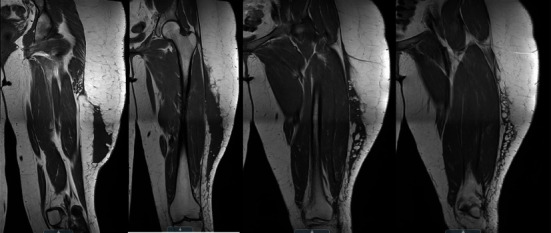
MRI T1 sequence of the left thigh approximately 1 month post-injury demonstrating large fluid collection with fat globules superficial to the deep fascia of the left thigh measuring 24.2 cm × 3.9 cm.
